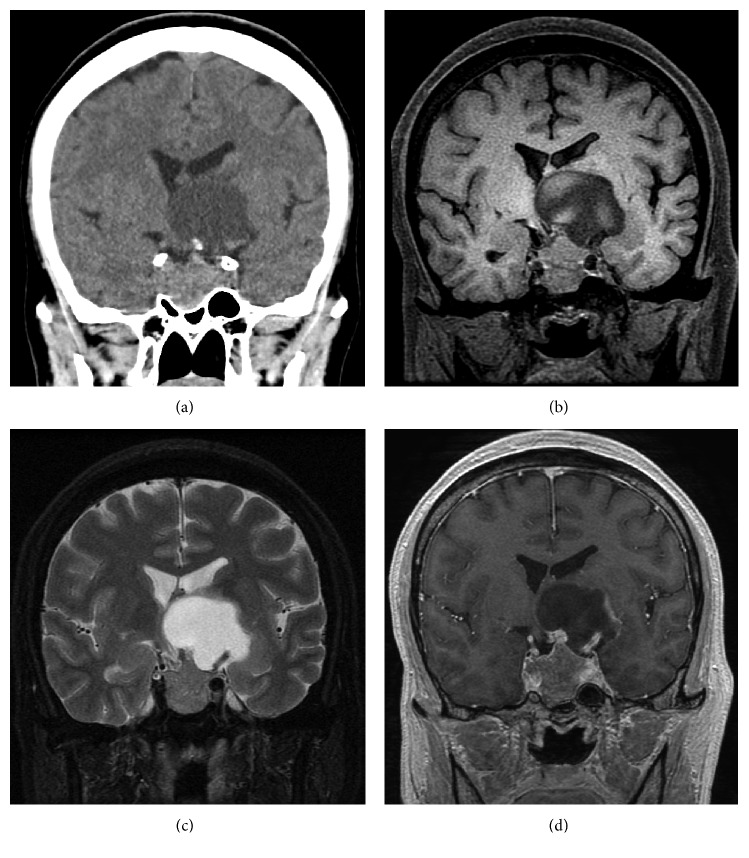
Coronal view of initial CT (a). Initial MRIs: T1-weighted (b), T2-weighted (c), and Gd-enhanced T1-weighted (d) coronal images.
